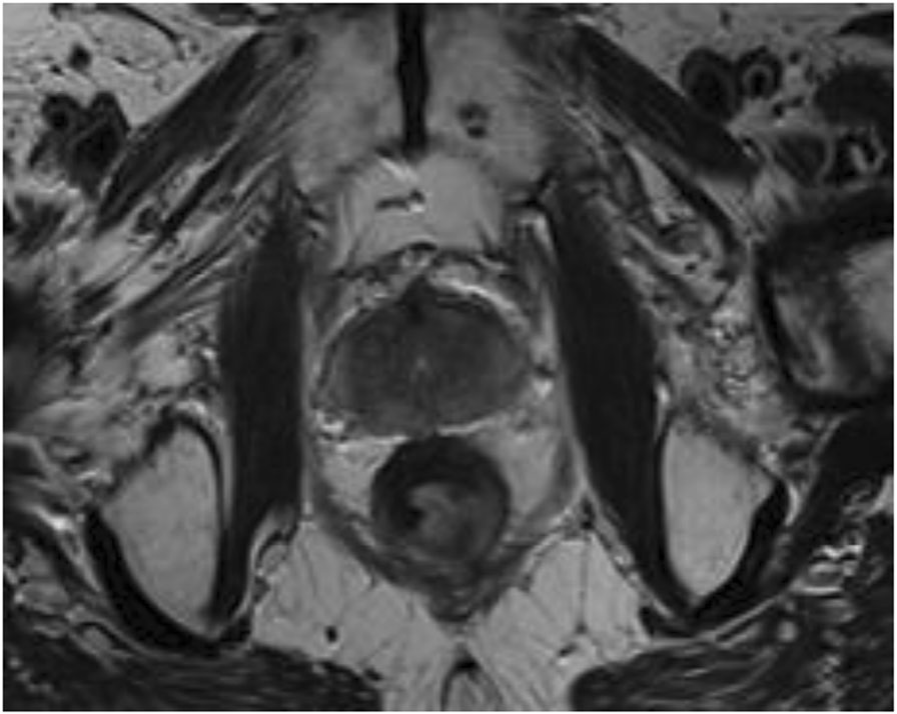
#### O3 Radiogenomics in rectal adenocarcinoma: associations between qualitative and quantitative MRI imaging features and genetic mutations
